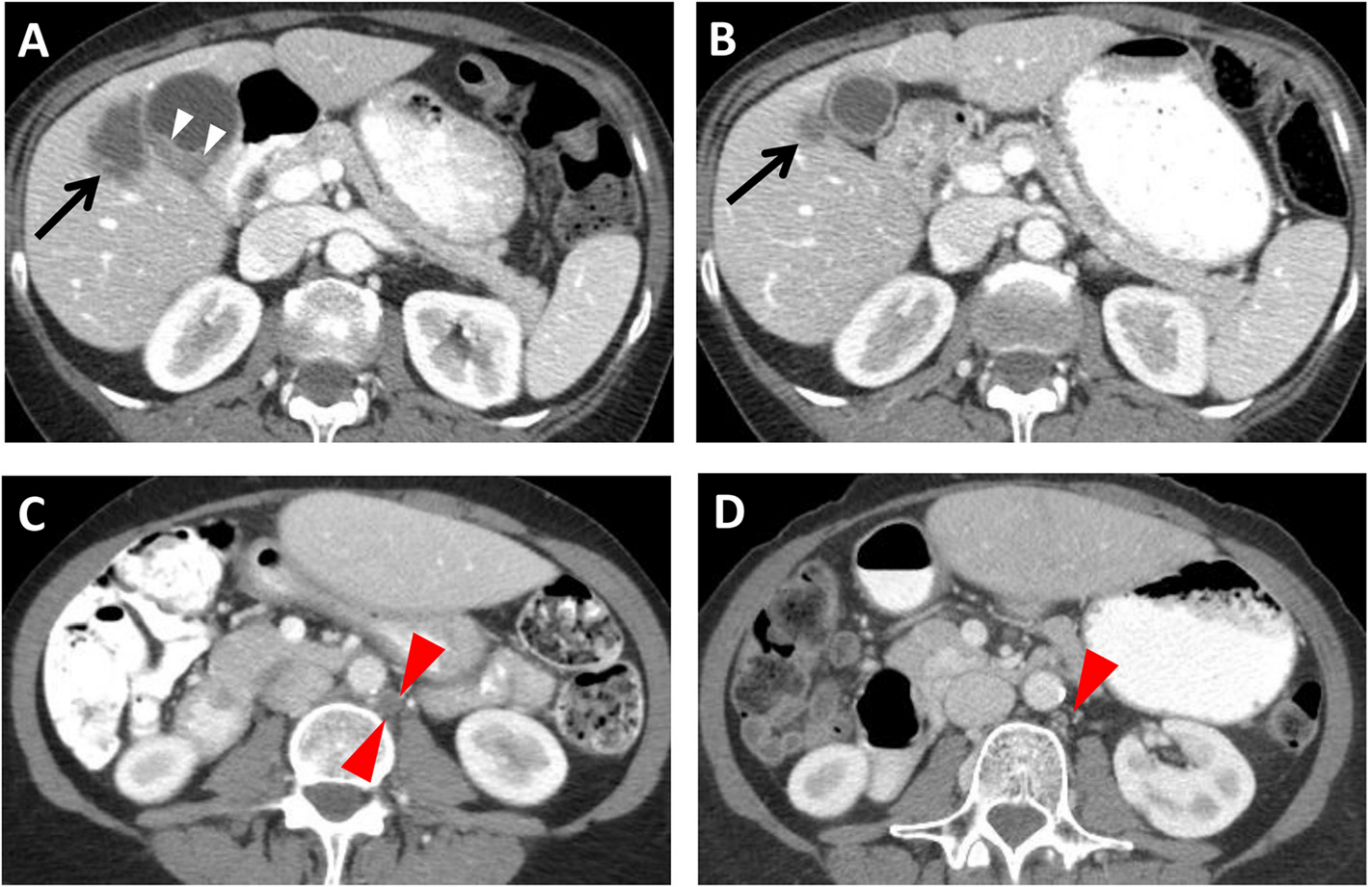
A 61-year-old female with gallbladder carcinoma invading the liver. Axial contrast-enhanced CT images demonstrate a a 2.4 × 1.3 cm polypoid mass (*small arrowheads*) in the gallbladder neck causing gallbladder obstruction. The mass directly invades the liver, with a 2.9 × 3.9 cm liver mass (*arrow*). b After 3 months of trastuzumab and FOLFOX, the polypoid gallbladder mass is no longer visualized, and the liver mass decreased to 1.2 × 1.1 cm (arrow). The patient was then treated with *en bloc* cholecystectomy and extended right hepatectomy, followed by capecitabine and trastuzumab for 7 months. c After 6 months of observation (14 months after surgery), the patient had small volume recurrence to retroperitoneal lymph nodes (*large arrowheads*). After treatment with FOXFOX and trastuzumab for 3 months, d a previously seen 1-cm retroperitoneal lymph node is nearly imperceptible
